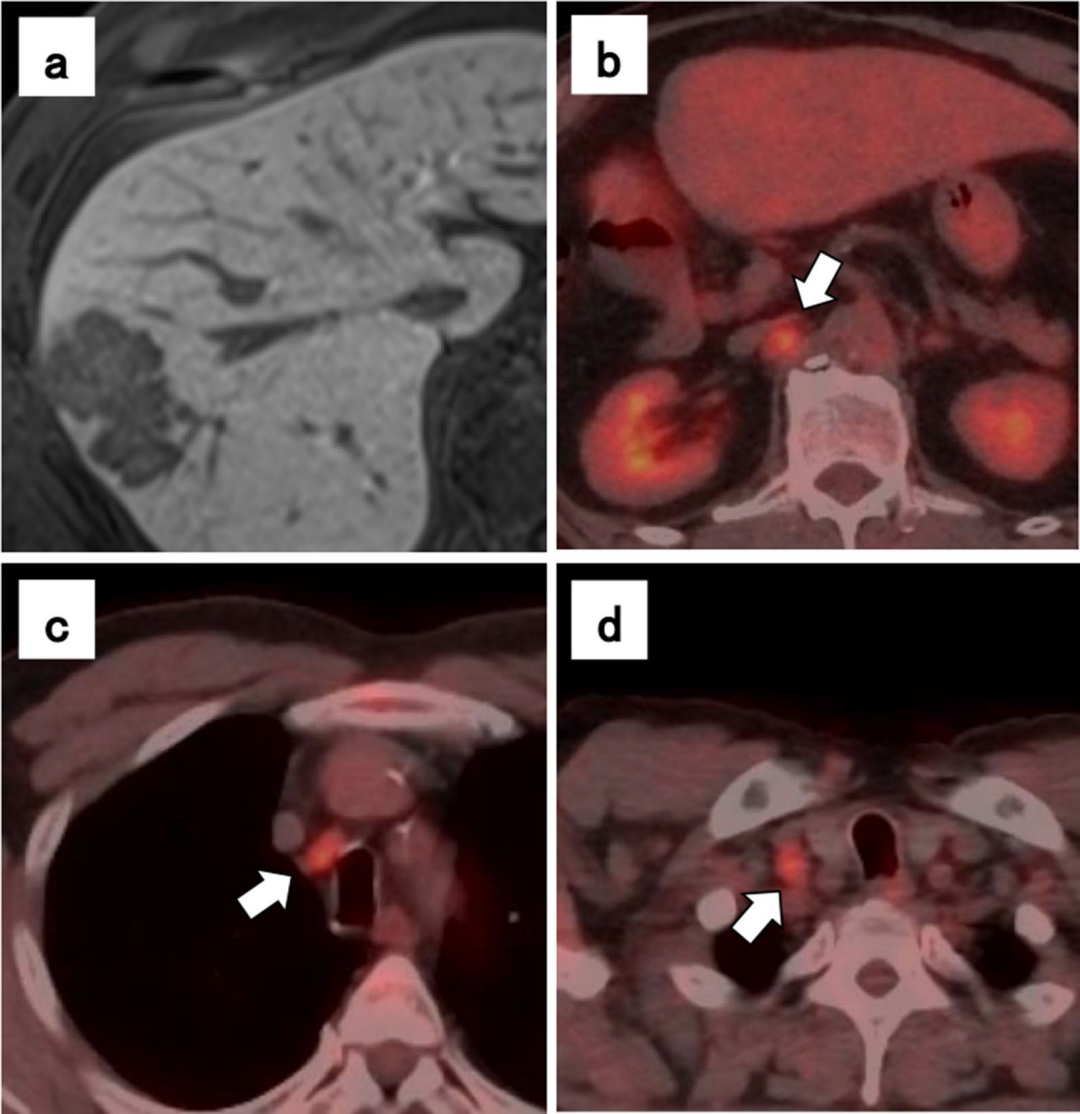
Hepatocellular carcinoma findings on magnetic resonance imaging, and lymph node metastasis on positron emission tomography–computed tomography (PET–CT) imaging. The tumor was located at the border of segments 8 and 7 of the liver (a). Lymph node metastasis seen on PET–CT, a subdiaphragmatic lymph node (b), an upper mediastinal lymph node (c), and a right cervical lymph node (d)
